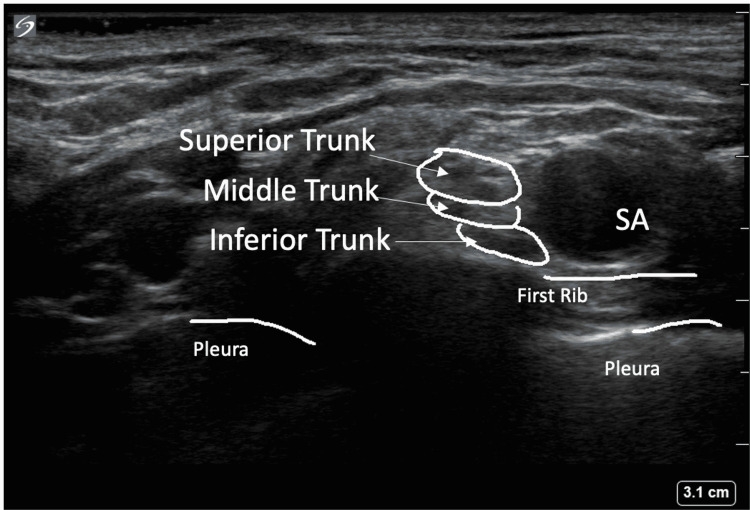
Ultrasound image of brachial plexus trunks at the supraclavicular level in relation to the subclavian artery, first rib, and pleura. SA: subclavian artery
